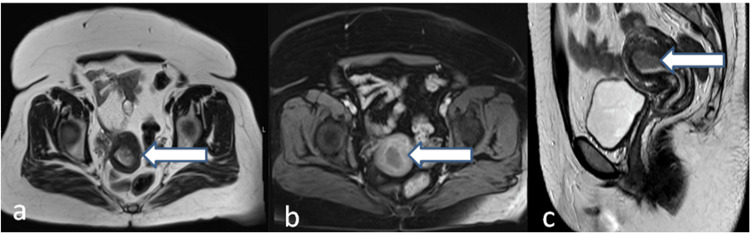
MRI of the pelvis showing thickened endometrium measuring 17 mm with intermediate signals on axial and sagittal T2WI (a and c). Minimal myometrial invasion (<50%) is seen on sagittal T2WI (c) with disruption of the junctional zone. Minimally enhancing endometrial tissue (b). No pelvic lymphadenopathy. MRI was staged as T1a N0. MRI: magnetic resonance imaging, T2WI: T2-weighted imaging
